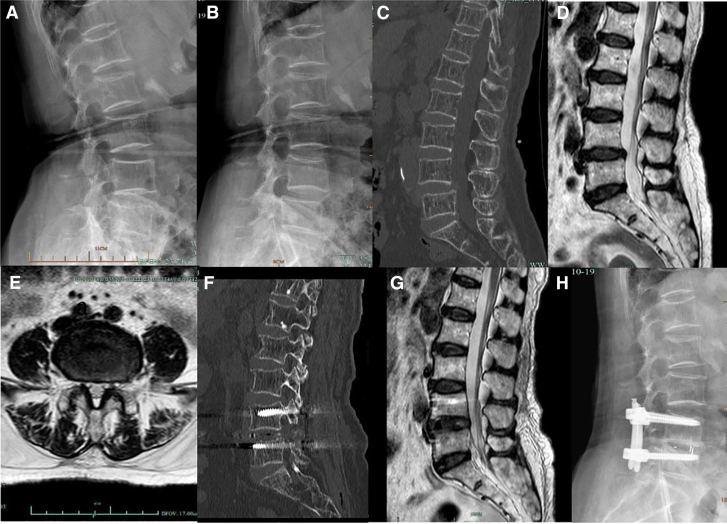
A 76-year-old female diagnosed with L4/5 spondylolisthesis and spinal stenosis, symptomatic for intermittent claudication after 100 meters of walking, was treated with L4/5 ULIF. Preoperative imaging revealed lumbar instability on flexion-extension radiographs (A, B) and L4/5 spondylolisthesis with spinal stenosis on CT (C) and MRI (D, E) scans. Postoperative CT (F) and MRI (G) revealed notable improvement, with a clear reduction in the L4/5 spondylolisthesis and significant alleviation of spinal stenosis. The 6-month follow-up radiograph (H) further indicated a successful outcome, demonstrating solid bony fusion at the L4/5 segment and stable fixation with no signs of screw loosening or migration. CT = computed tomography, ULIF = unilateral biportal endoscopic lumbar interbody fusion.
